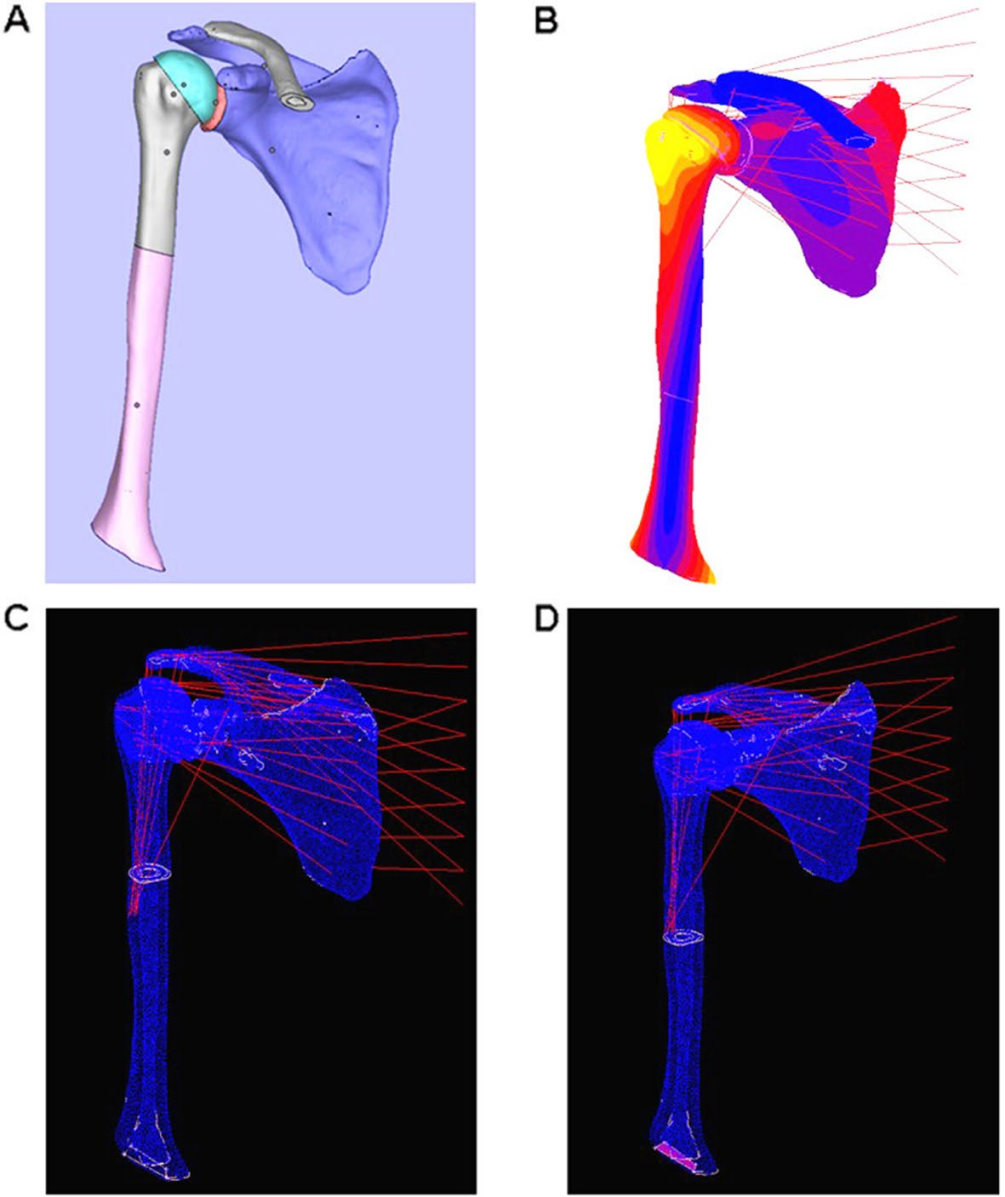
FE model construction. A the stereolithography model construction. Based on the CT data of the normal shoulder of a 31-year-old female, the stereolithography model was constructed by MagicRP and Geomagic software. B the reconstructed muscles model. The lines represented the spring. C postoperative malrotation model of humeral shaft fracture with osteotomy above the insertion of deltoid. D postoperative malrotation model of humeral shaft fracture with osteotomy below the insertion of deltoid
